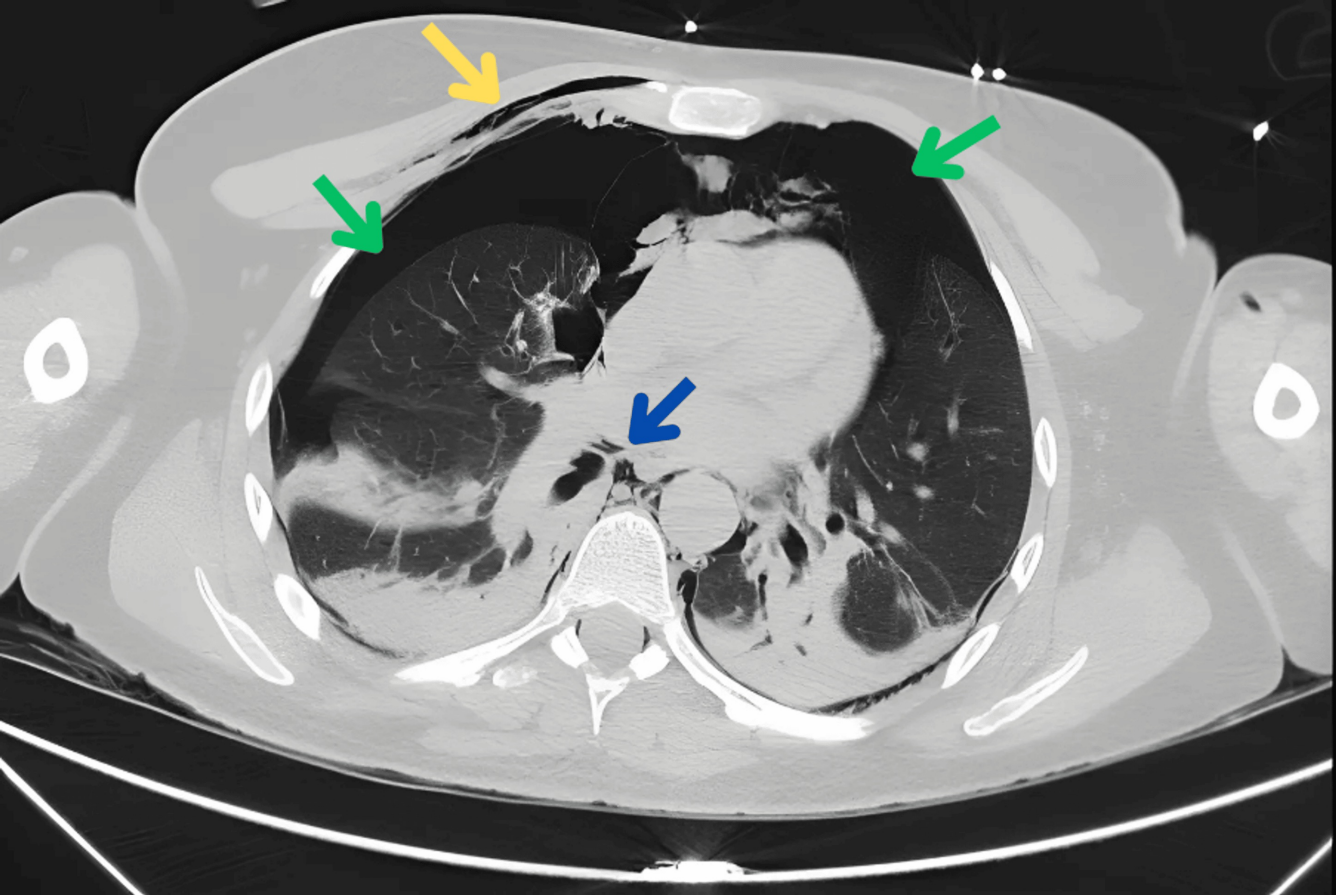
CT image (axial): bilateral pneumothorax. Blue arrow: pneumomediastinum; green arrow: pneumothorax; yellow arrow: subcutaneous emphysema
